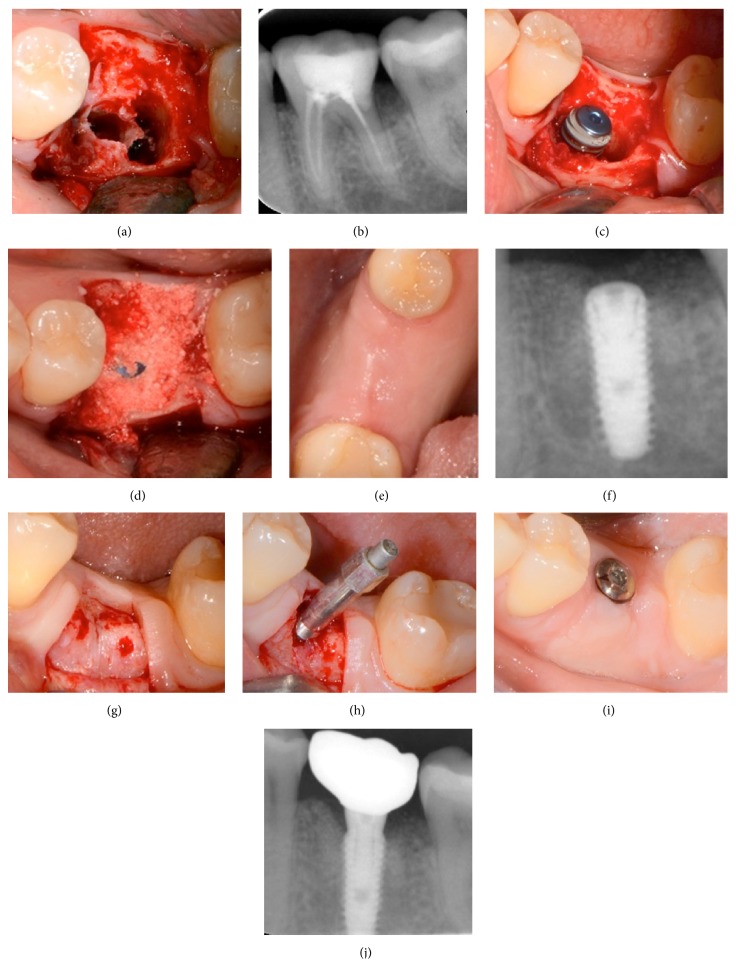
Case  1: a 47-year-old woman with crown and root fracture in the left mandibular first molar. (a) Clinical view of the site after thorough debridement of the socket. (b) Periapical X-ray of the nonrestorable tooth. (c) Implant placement at the correct 3D positioning. ISQ reading was 48. (d) Grafting with *β*-TCP/CS (Ethoss). (e) Clinical view after 10 weeks. (f) X-ray 10 weeks after implant placement and grafting showing the consolidation of the grafting material around the implant and new bone formation over the implant head and towards the adjacent interproximal heights of bone. (g) At reentry the site is filled with regenerated bone. Note the head of the implant covered by newly formed bone. (h) After removing the supernatant newly formed bone with a round burr implant stability is assessed (ISQ measurement: 78) revealing a significant increase through the 10-week healing period. (i) Maturation of the soft tissues 2 weeks after placement of the healing abutment. (j) X-ray 9 months after loading.
